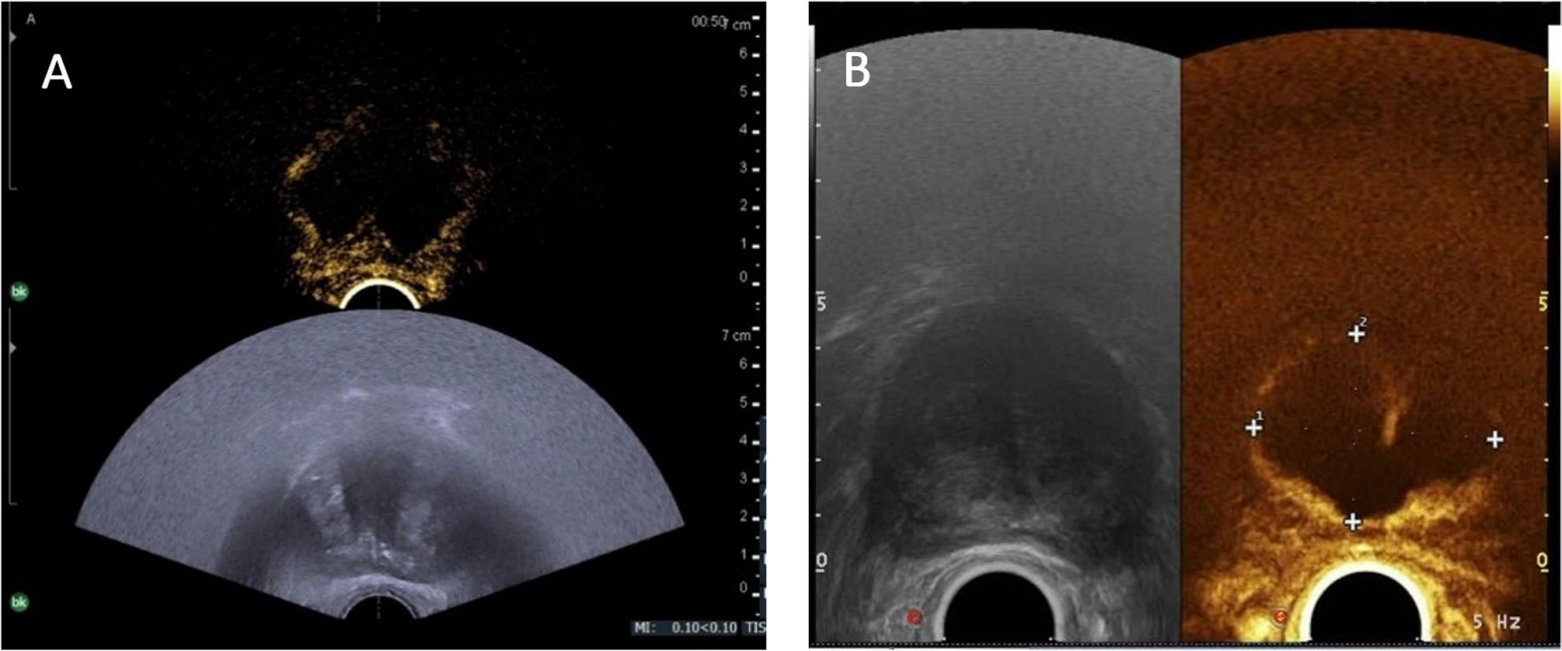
Immediate contrast-enhanced ultrasound of the prostate after modified water vapor thermal therapy (A). Contrast-enhanced ultrasound of the prostate at 1 month after modified water vapor thermal therapy (B)
